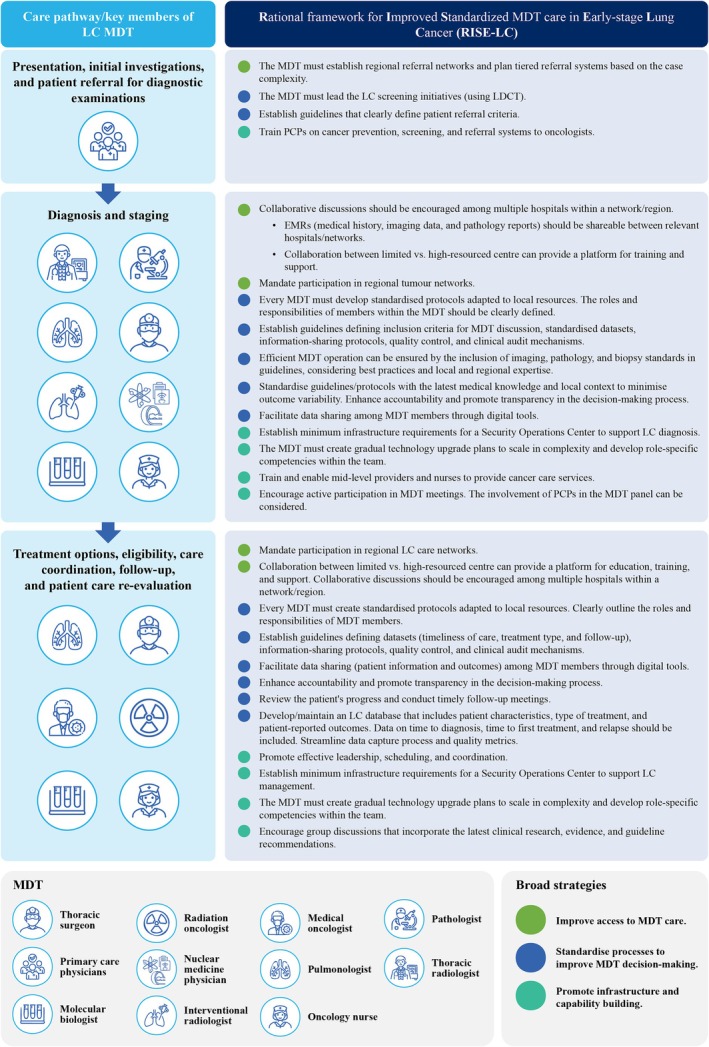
Key strategies for strengthening MDT care for ESLC in LMICs and emerging economies. EMR, electronic medical record; LC, lung cancer; LDCT, low‐dose computed tomography; LMICs, low‐ and middle‐income countries; MDT, multidisciplinary team; PCP, primary care physician.
